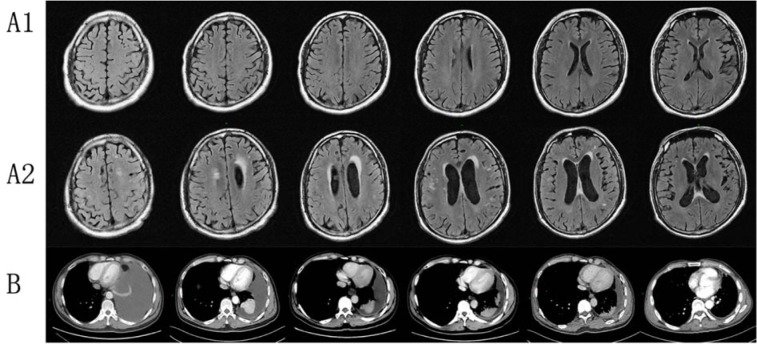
Clinical images of Case 1. (A1) Brain MRI with contrast of brain for patient 1 displaying normal status before EGFR-TKI therapy; (A2) Brain MRI showed increased ischemic foci and lacunar infarction, serious encephalatrophy, and demyelination after EGFR-TKI therapy; (B) Chest CT with contrast of patient 1 showed gradually decreasing pleural effusion in EGFR-TKI treatment.
